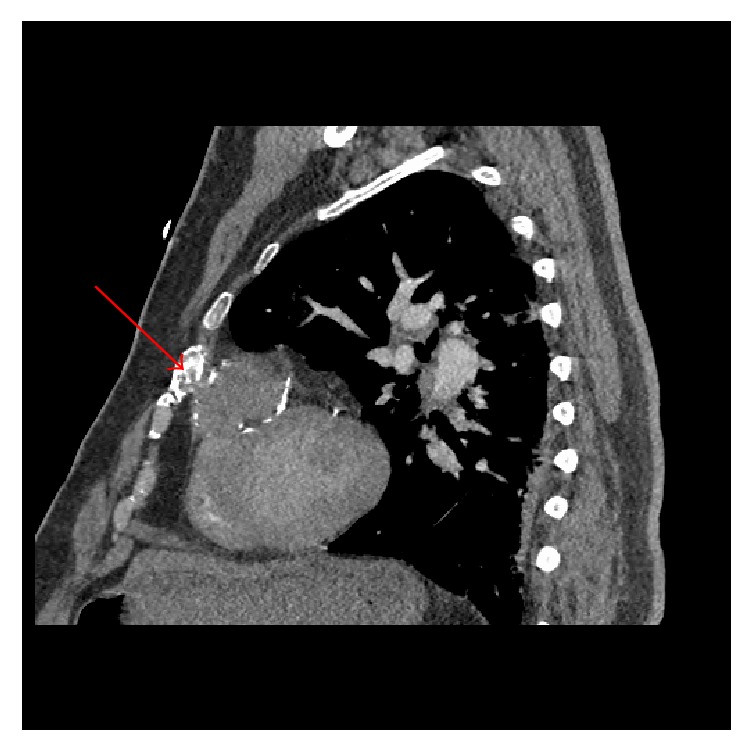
CT pulmonary angiogram. An old healed rib fracture anterior to the pseudoaneurysm (red arrow).
